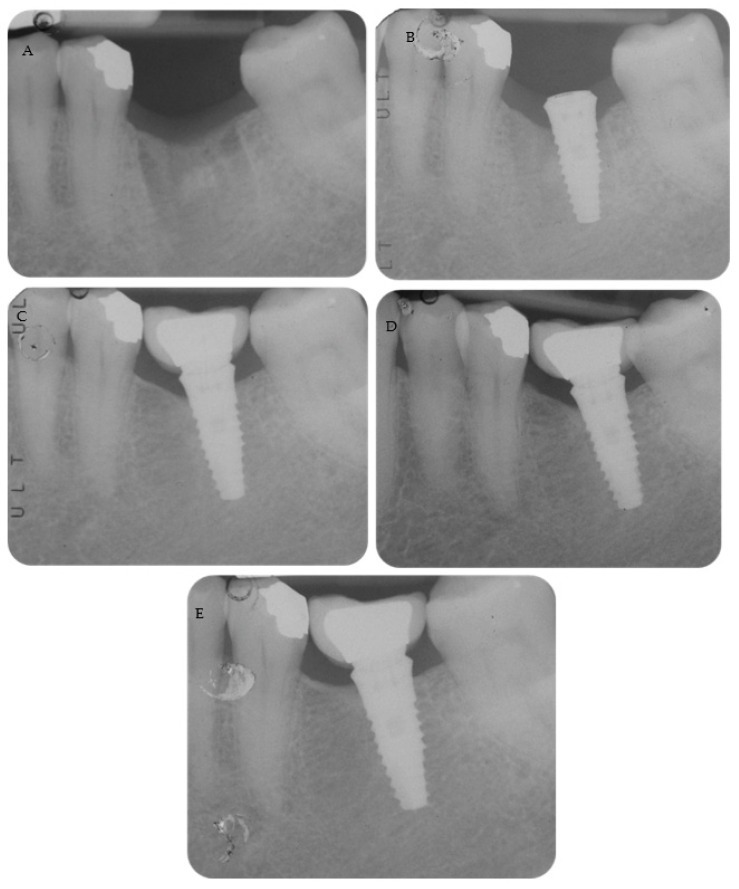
(A) Early timing placement to rehabilitate a single edentulous ridge. Tooth was extracted 2 months before for a root fracture. (B) Implant placement with a flapless approach. Please note that the neck was positioned at tissue level. (C) Periapical radiograph after abutment and definitive crown cementation. (D) Follow-up at 36 months and (E) 48 months showed MBL stability around the implant.
